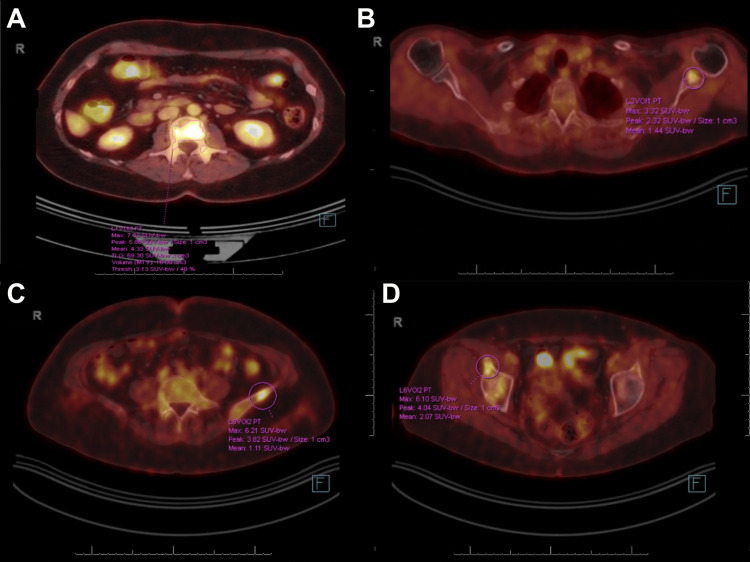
Axial fluorodeoxyglucose positron emission tomography/computed tomography images demonstrating metabolically active sclerotic osseous lesions in a patient with metastatic breast cancer. Focal hypermetabolic activity is seen in the L2 vertebral body (A, maximum standardized uptake value (SUVmax): 7.82), left glenoid (B, SUVmax: 3.32), left iliac bone (C, SUVmax: 6.21), and right iliac bone anterior to the hip (D, SUVmax: 6.10). These findings are consistent with osseous metastatic disease.
